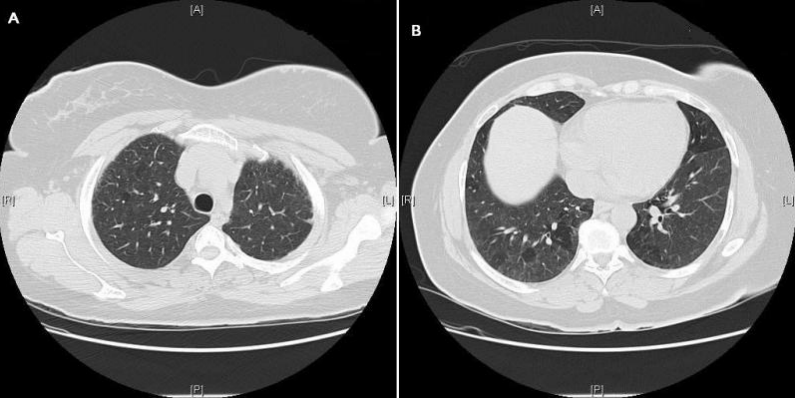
Case 3. (a) HRCT scan obtained from the upper lung zones shows interlobular reticular opacities and patchy ground-glass attenuation. (b) HRCT scan at the basal portion of the lungs showing multilobular regions of air trapping.
